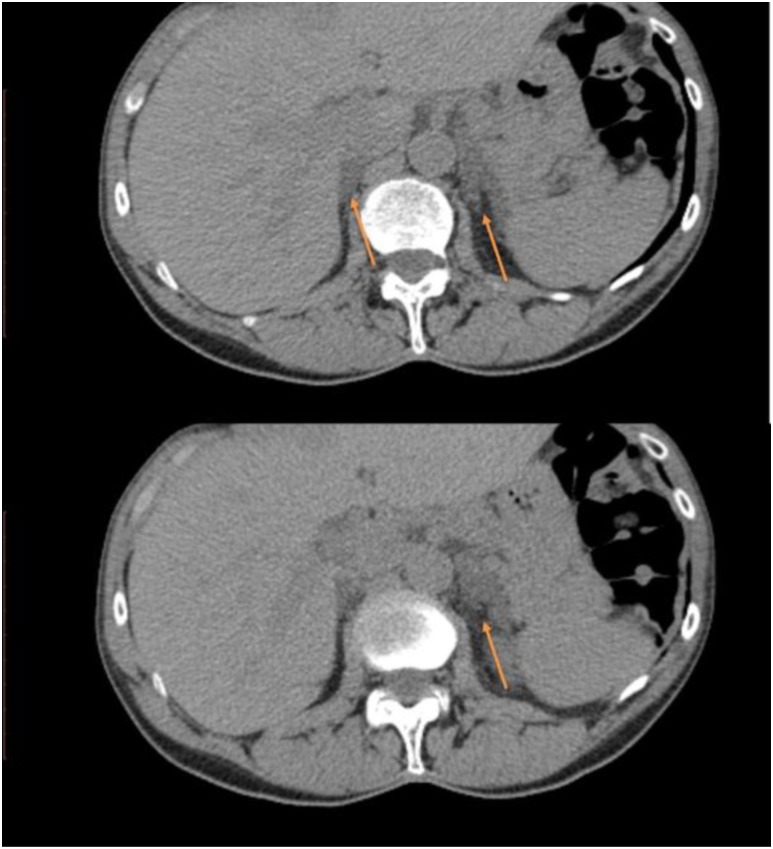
CT images from case 1 showing an enlarged right gland and nodular hyperplasia on the left gland (arrows).
